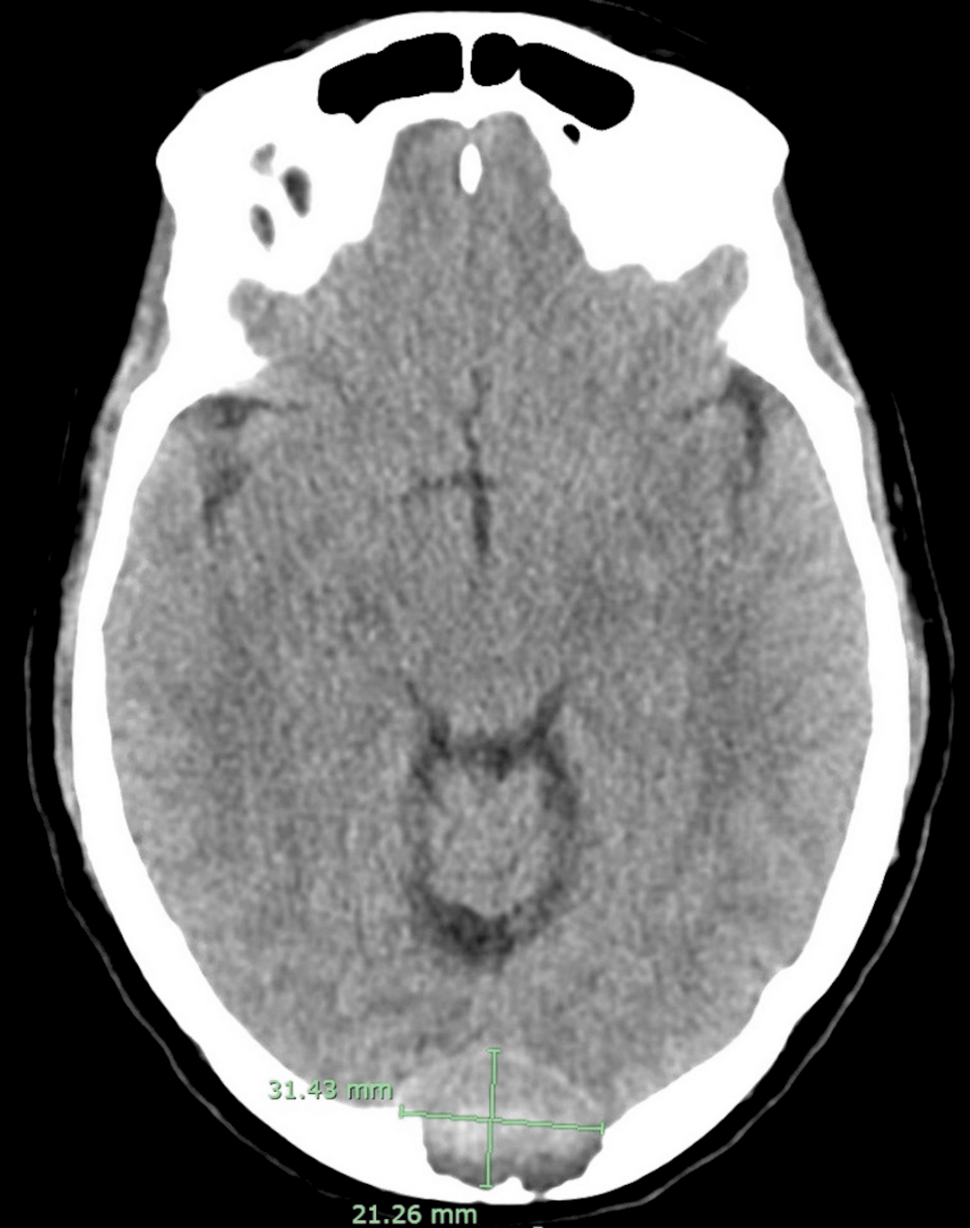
CT shows a heterogeneous extra-axial mass demonstrated at the level of the torcula and appeared to be displacing the dural venous sinuses anteriorly. The mass causes nearly full-thickness destruction of the occipital bone. The mass measures 2.1 x 3.1 x 2.8 cm in diameter. CT: computed tomography
